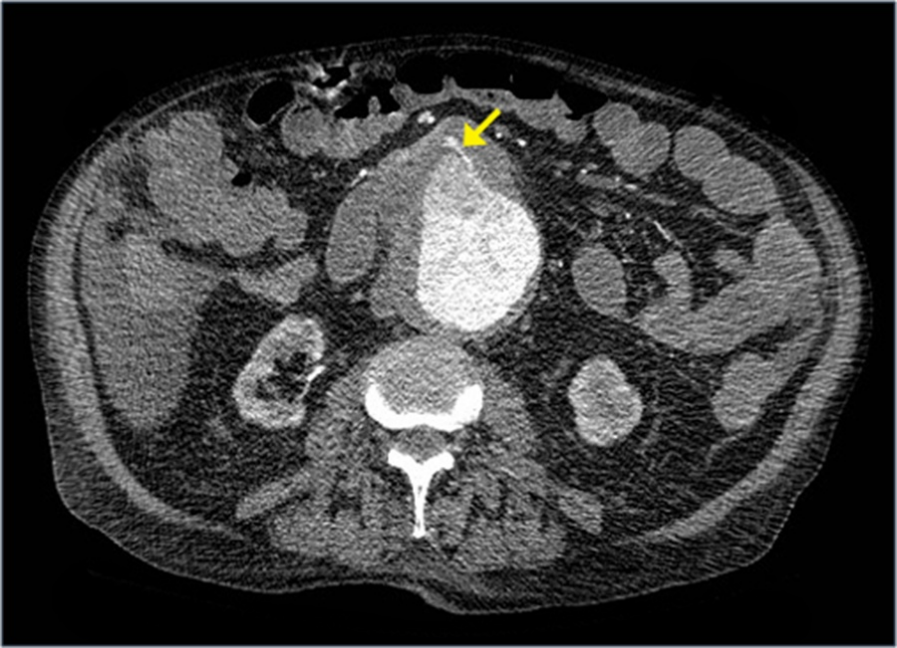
Primary Aortoenteric Fistula (PAEF) Axial contrast-enhanced (IV only) CT image, obtained a few slices inferior to Figure 1, shows an abdominal aortic aneurysm (AAA) measuring up to 11 cm and presence of a fistula leading into the duodenum (arrow).
